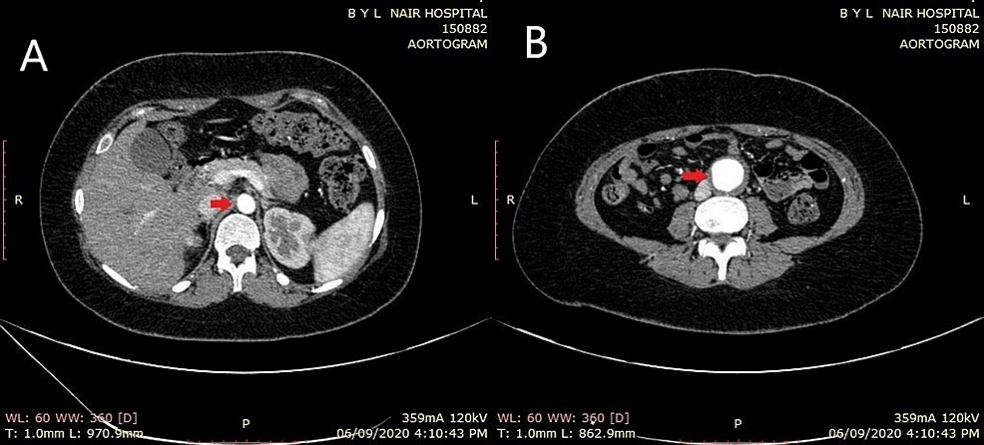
CT aortogram (transverse section) CT images showing dilated infrarenal aorta (red arrow) measuring 3 cm X 3.2 cm (B) as compared to the normal suprarenal aorta (red arrow) measuring 1.2 cm X 1.4 cm (A)
